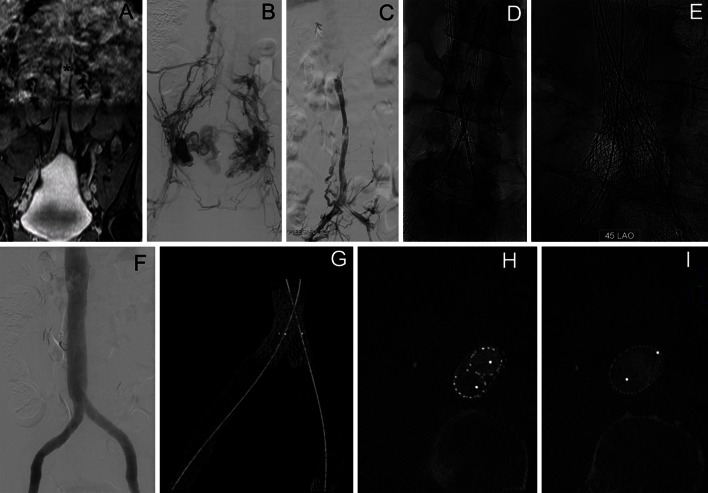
Confluence stenting with balloon-expandable stents (BECS). A pre-operative MR venography shows an IVC remnant (*arrow*). Notice the post-thrombotic scarring in the external iliac vein (*arrowhead*). *Asterisk* aorta. B angiography shows severe obstruction of bilateral iliac veins and no filling of the IVC. C after iliac recanalization angiography from the level of the confluence shows a long stenosis in of the IVC. D and E positioning and deployment of two balloon-expandable stents. F completion angiography shows excellent flow from both iliac limbs through the newly constructed iliac confluence and IVC. G–I Conebeam CT reconstructions showing perfect positioning and expansion of the stents at the confluence and IVC
